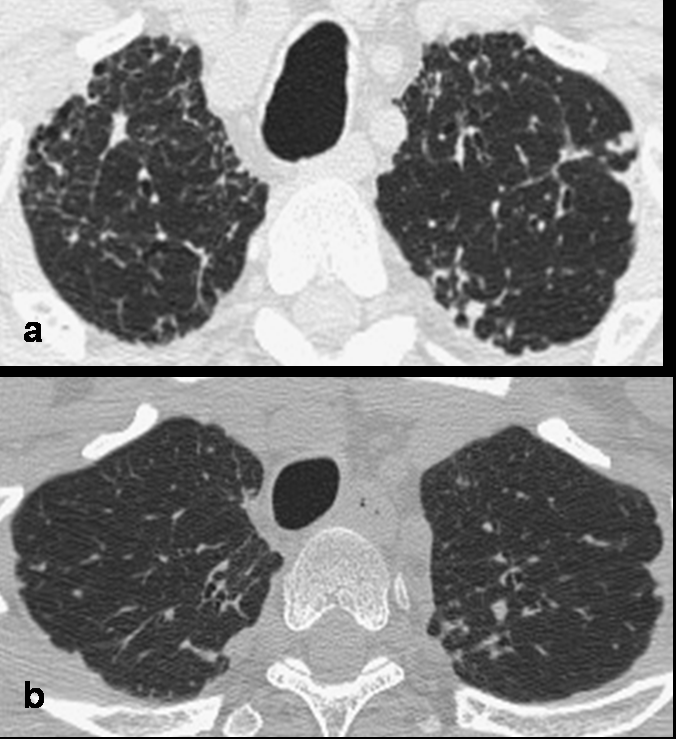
CT scan of apical region in both patients (a: patient n°1 and b: patient n° 2) shows a diffuse thickening of pleuroparenchymal interface, suggesting IPPFE.
